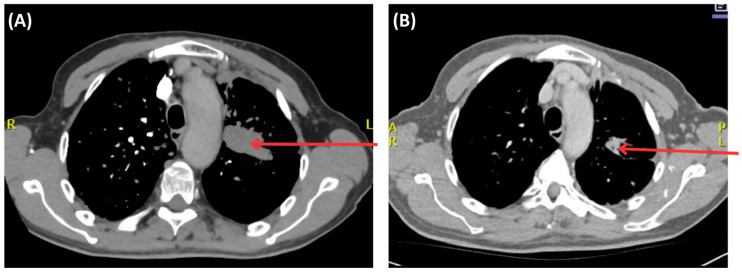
CT scan of the chest: Pre-treatment CT scan (15 August 2024) at baseline showing dominant left upper lobe/infrahilar mass [red arrow] with innumerable millimetric metastatic nodules bilaterally (A); most recent CT scan (5 September 2025) showing the residual focal opacity in the left upper lobe [red arrow] has decreased in size with some of the cavitary nodules being smaller, showing overall partial treatment response with osimertinib (B).
